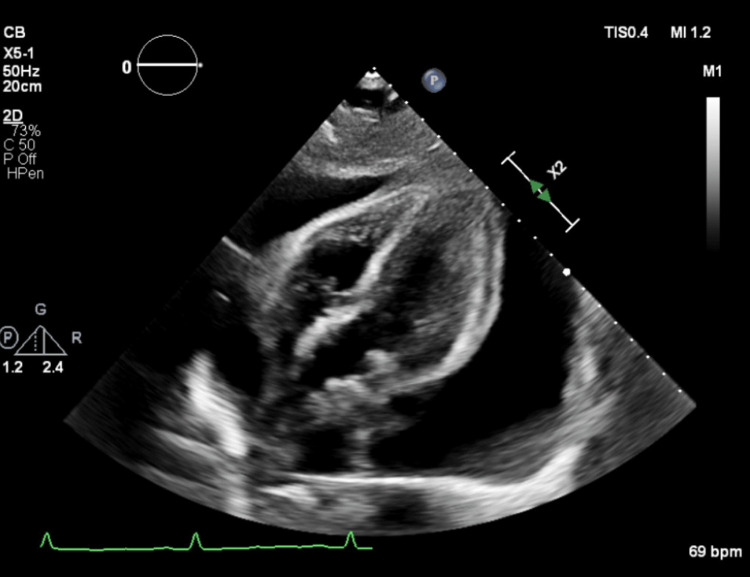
Apical four-chamber echocardiographic view demonstrating a large circumferential pericardial effusion with right atrial systolic collapse suggestive of early tamponade physiology, without right ventricular diastolic collapse.
